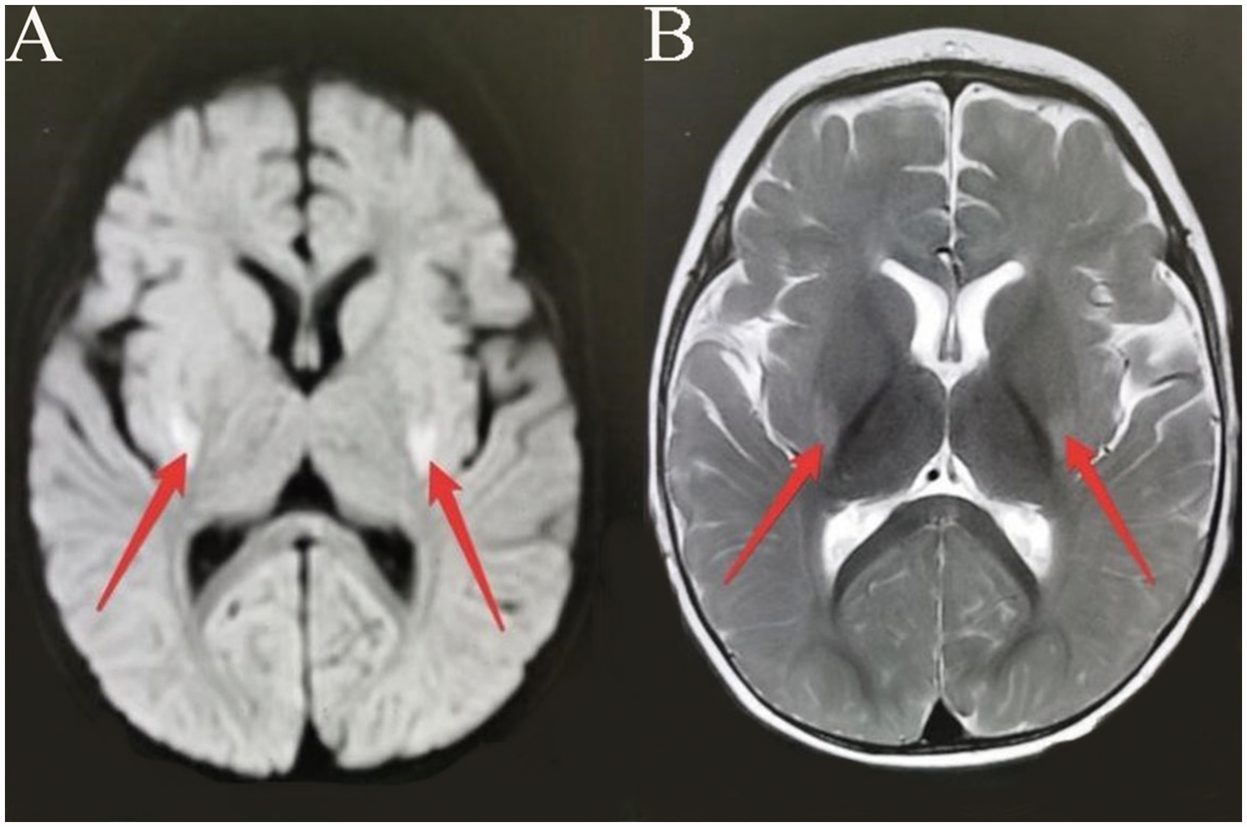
(Patient 2) Brain MRI. (A) T1-weighted image: bilateral posterior putamen hyperintensity. (B) T2-weighted image: bilateral posterior putamen hyperintensity.
